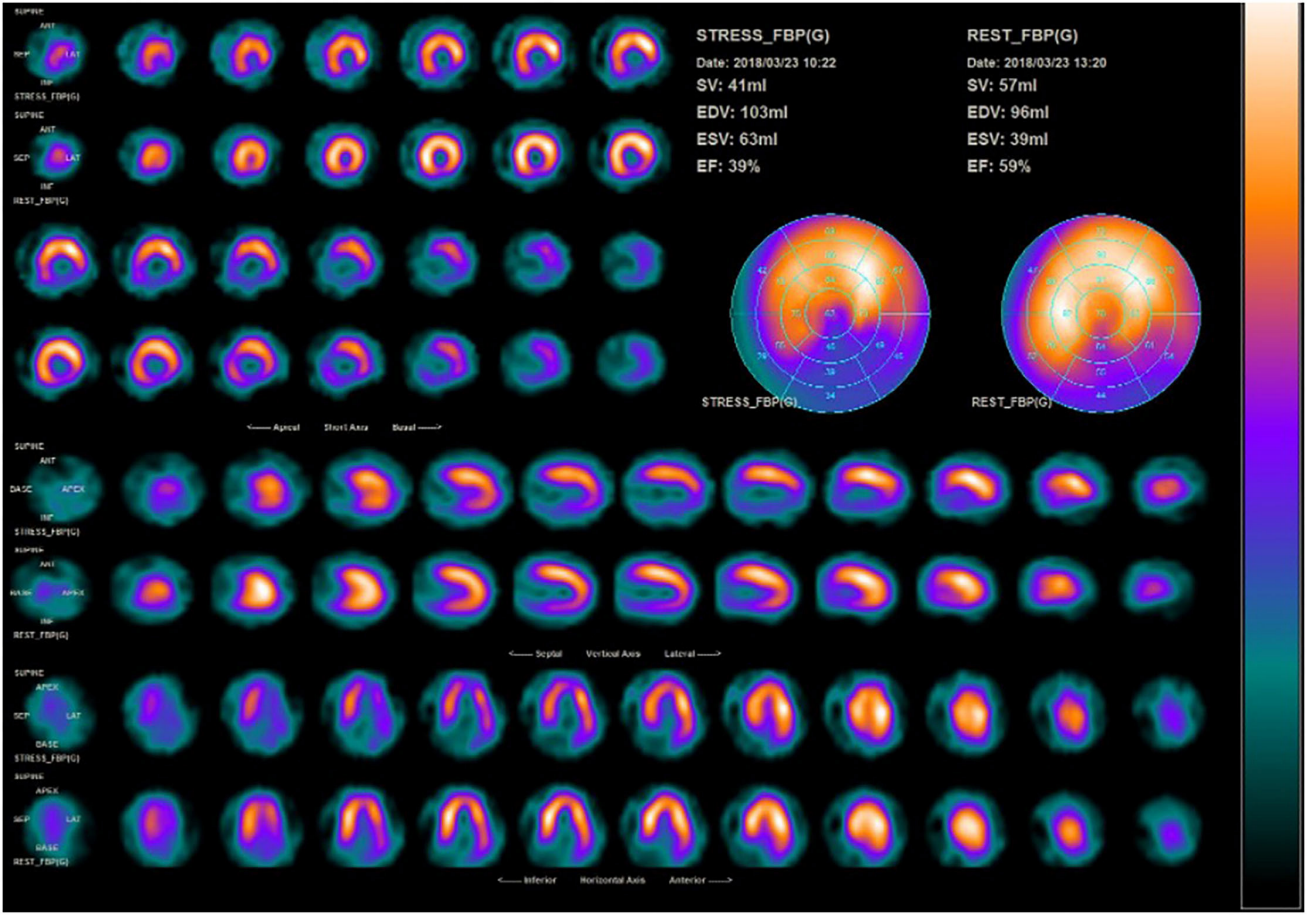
SPECT examination of patient 1 before ESMR therapy.
